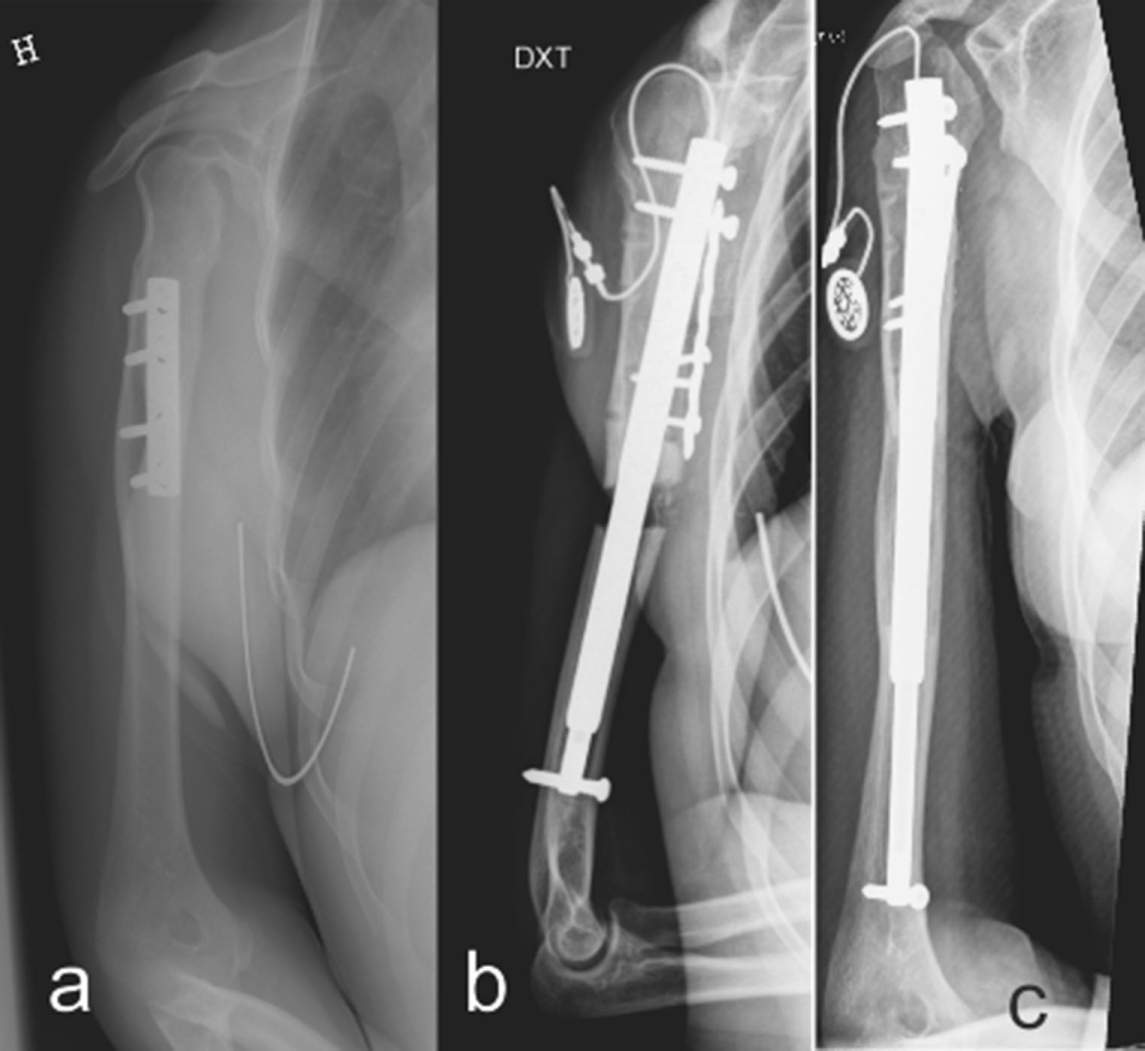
a Preoperative radiograph of 19-year-old patient T.K. with 65 mm shortening of the right humerus after Erb–Duchenne-type obstetric palsy. The patient had at the age of 12 years an external rotation osteotomy fixed with a plate. b Follow-up radiograph after lengthening has been initiated by the inserted FITBONE. After the previously inserted plate was removed at the time of FITBONE insertion a cortical defect existed. In order to securely lock the proximal part of the nail, a new plate was fixed in good cortical bone distally and one of the proximal locking screws were inserted through a plate hole. c Radiograph after consolidation of 6-cm lengthened humerus
